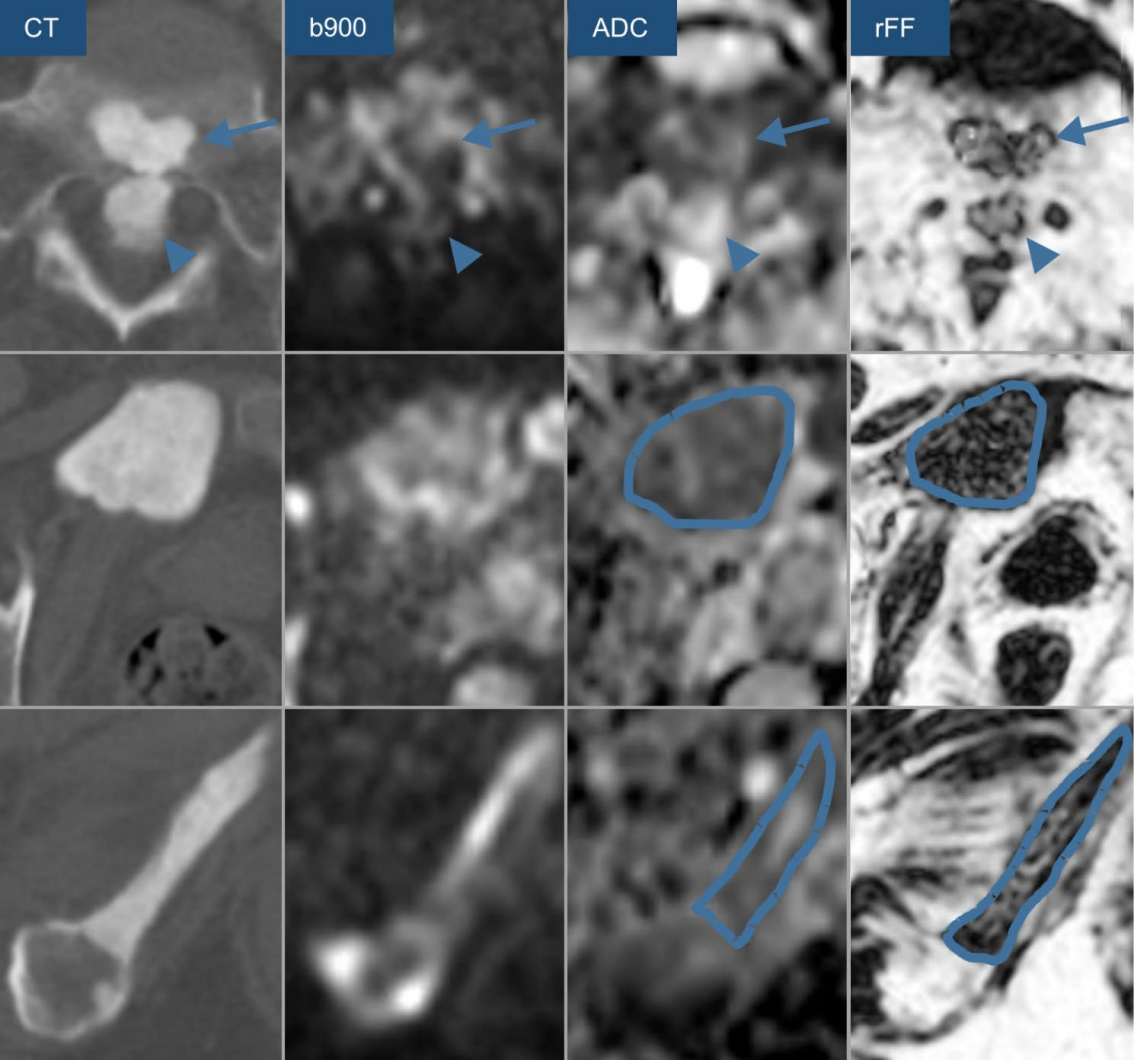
CT-guided bone biopsy target lesion selection process based on multiparametric bone MRI, considering high b-value DWI (b900) signal intensity (SI) ADC and rFF in a metastatic castrate resistant 74-year-old prostate cancer patient with multifocal sclerotic bone metastases, CT images for comparison. The anterior metastasis in the first row (arrow) shows low DWI SI, the posterior lesion (arrowhead) displays high ADC (> 1100 µm^2^/s) and high rFF (> 20%) and was thus not fit for biopsy. The pubic bone metastasis in the second row shows high DWI SI, ADC < 1100 µm^2^/s and rFF < 20% and was biopsied, biopsy provided sufficient malignant tumor cells for next-generation genomic sequencing. The ischial metastases in the third row displays high DWI signal, an ADC < 1100 µm^2^/s, but an rFF of 31% and was thus not considered for biopsy
